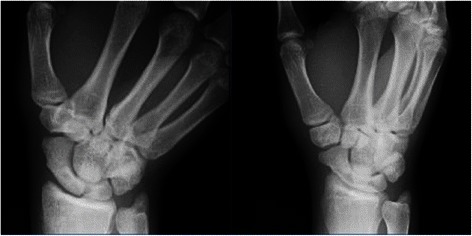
Radiographs obtained at the first visit show a nondisplaced scaphoid waist fracture through the carpal scaphoid with slightly increased bone density
